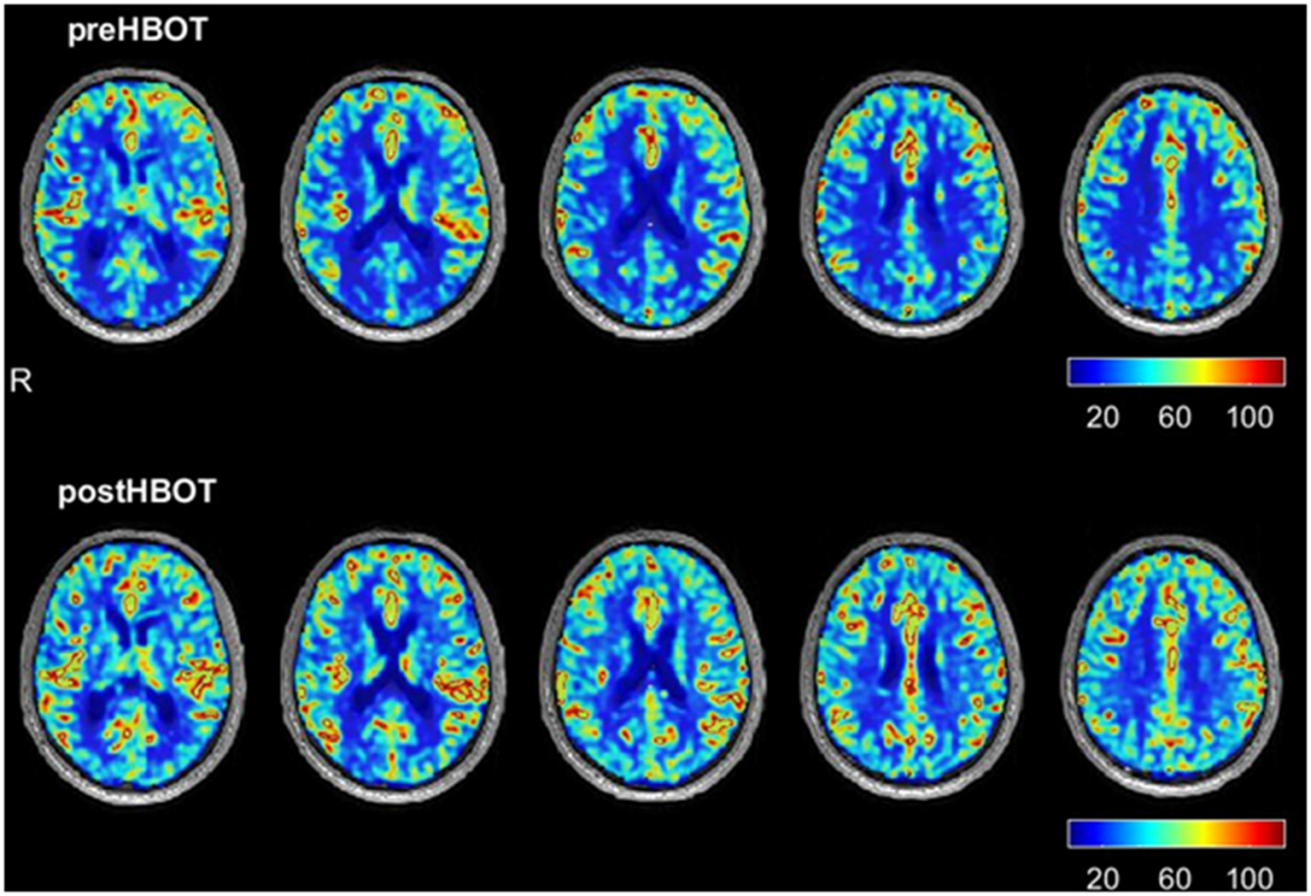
Brain MRI perfusion changes (DSC).
